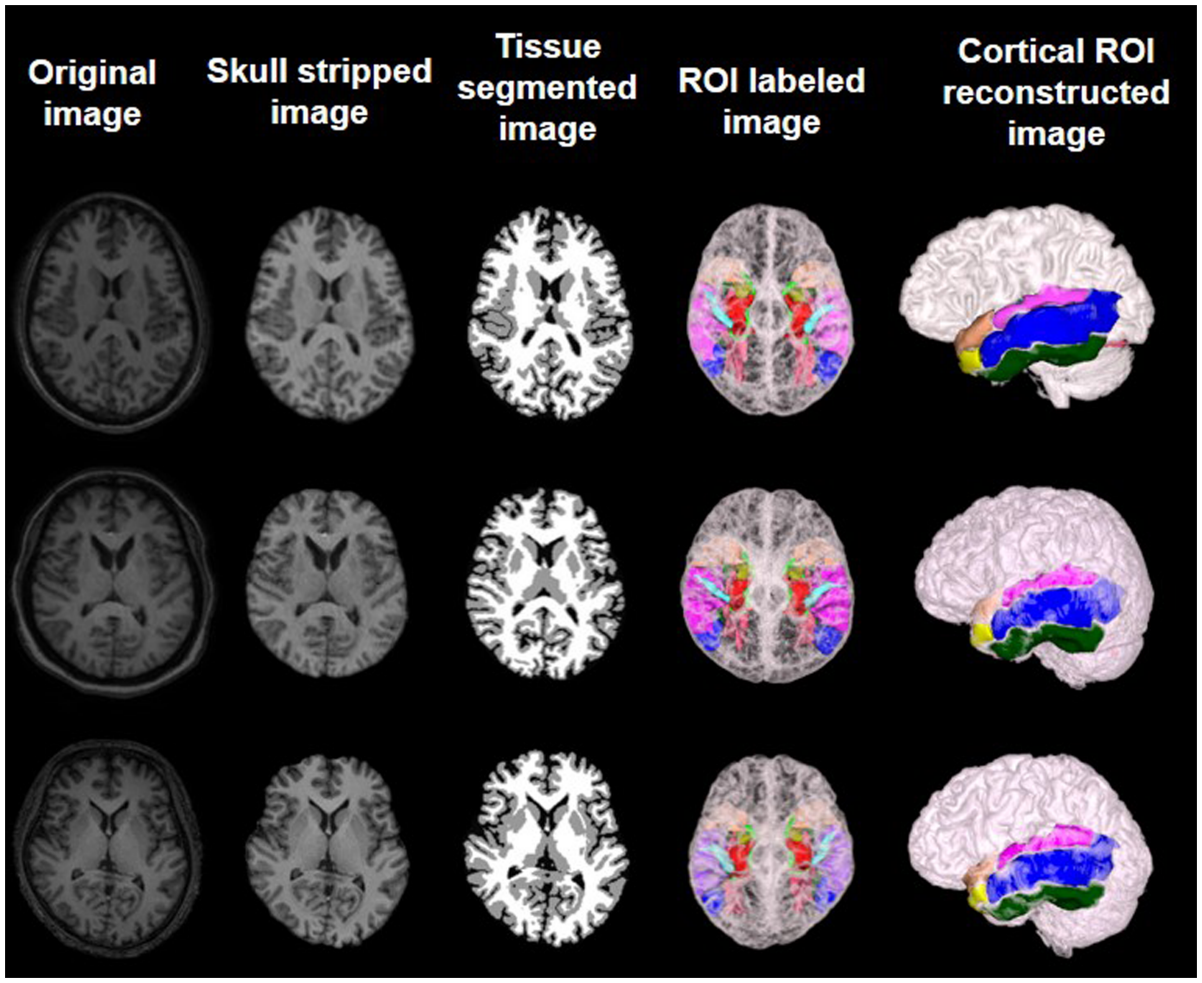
Preprocessed MR images and feature extraction on brain labeled and cortical reconstructed MR images of three different subjects.
